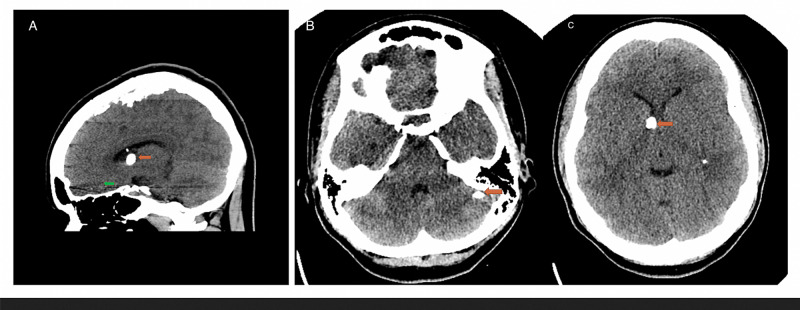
(A) CT, sagittal view, showed calcification in the temporal lobe under the knee of the corpus callosum (red arrow), and also shows frontal lobe hypodensity (green arrow). (B) CT, transversal view, shows calcification in the right cerebellar hemisphere (red arrow). (C) Transversal view shows a calcification at the level of the posterior horn of the left ventricle (red arrow)
